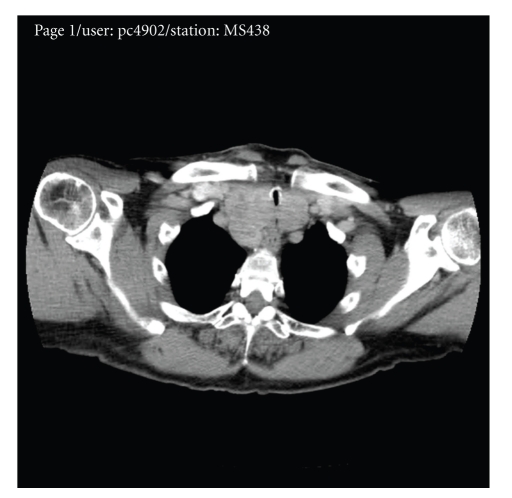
CT scan showing the retrosternal goitre compressing the trachea.
